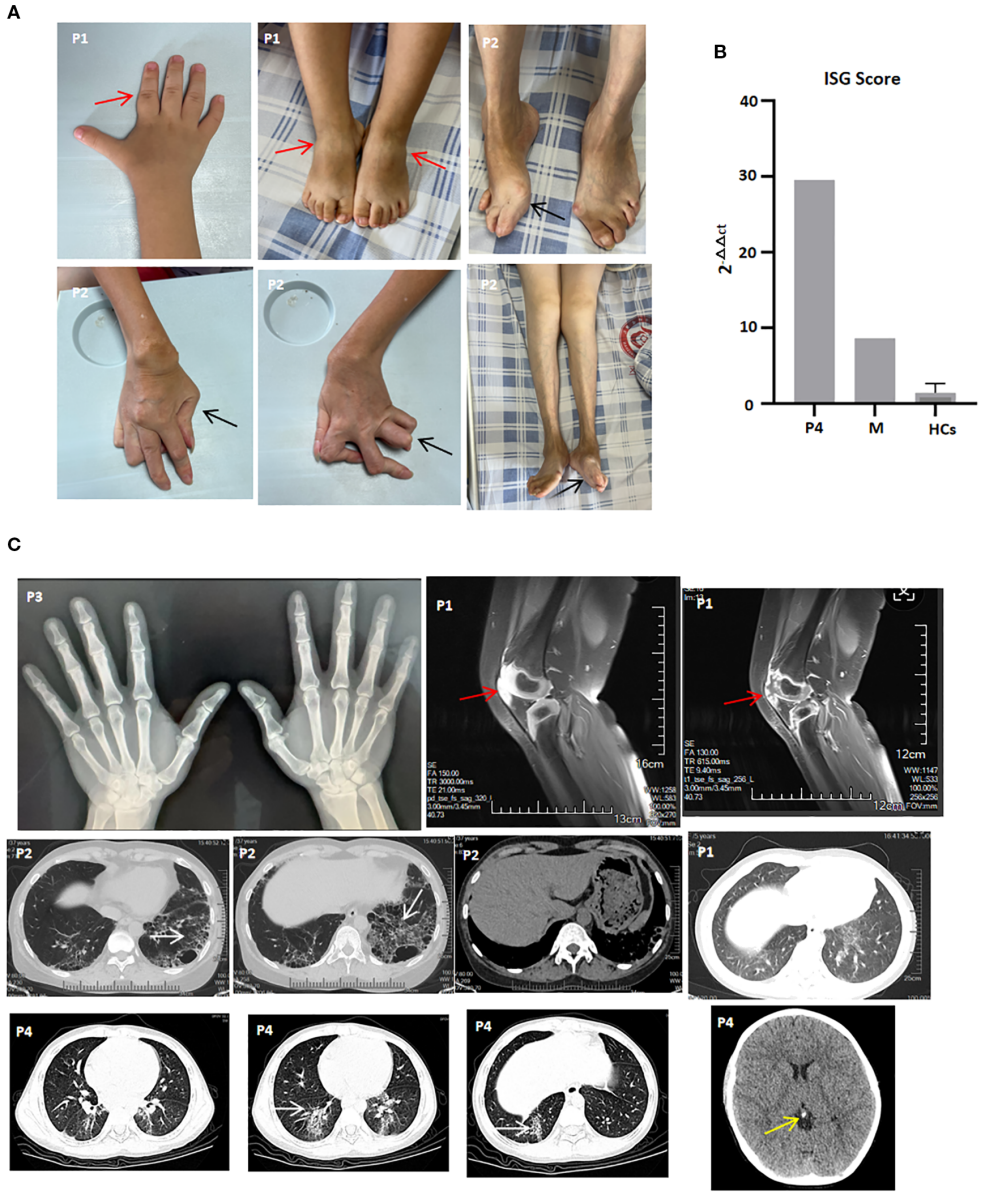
Clinical features and imaging manifestations of patients with the *UNC93B1* c.1007G>A p.R336H heterozygous variation. (A) Active arthritis (P1) and joint sequelae (P2). Red arrow indicates joint swelling, and black arrow indicates joint deformity; (B) ISG score of P4, his mother (M) and healthy controls (HCs), shown as one representative result of two repeated experiments; (C) X-ray of both hands (P3), knee MRI (P1), lung CT (P1, P2, and P4), and brain CT (P4). White arrow indicates interstitial lung diseases, red arrow indicates joint effusions and synovitis, and yellow arrow indicates calcification.
